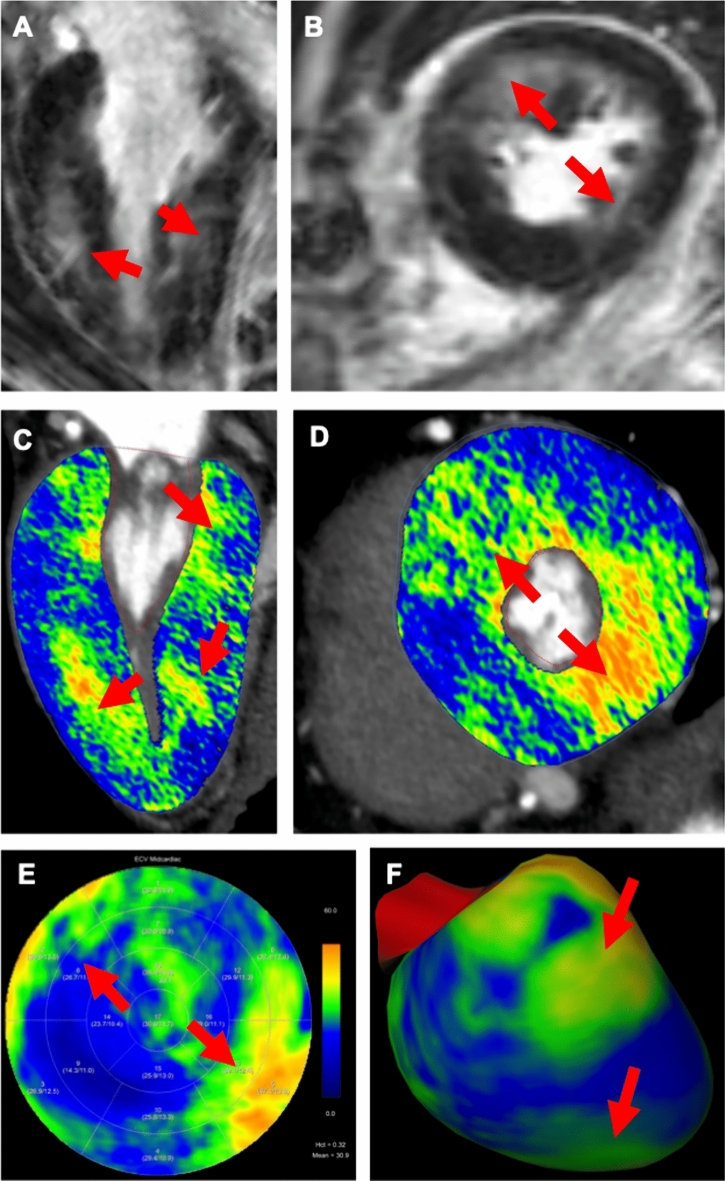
Sixty nine-year-old male patient with hypertrophic cardiomyopathy. Red arrows indicate typical basal accentuated left ventricular hypertrophy with late gadolinium enhancement at right ventricular insertion points in cardiac MRI in A two-chamber and B short axis views. Corresponding extracellular volume elevations are shown also on C two-chamber and D short-axis views in CT, additionally represented in the 16-segment E polar map and on a F three-dimensional heart model
